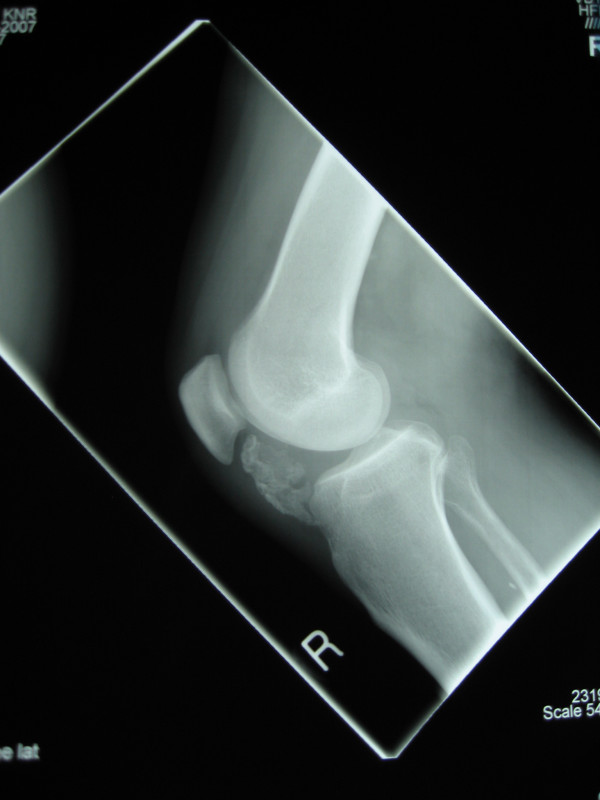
Lateral radiograph of the knee demonstrating ossification in the peritendinous tissues.
